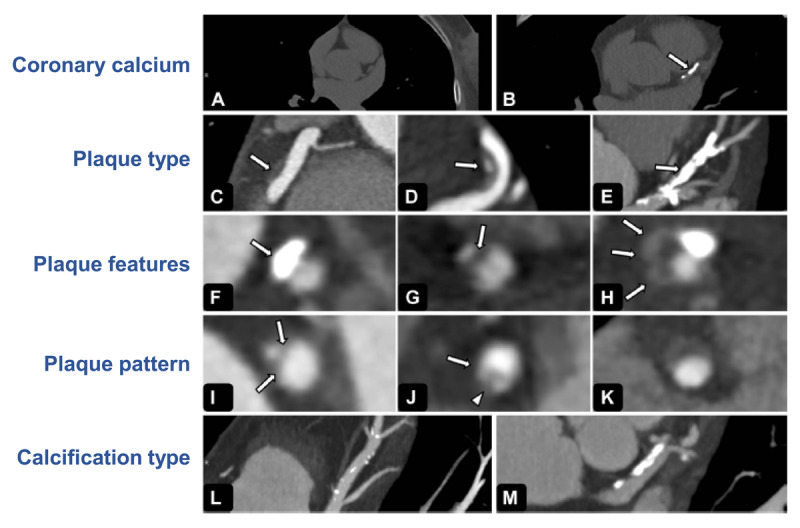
Plaque analysis by CCTA. To assess the (A) absence or (B) presence of coronary artery calcium. Plaque type is classified as noncalcified (C), partially calcified (D), or calcified plaque (E). Plaque features are classified as positive remodeling (panel (F) shows external remodeling of calcified plaque), (G) low-attenuation plaque, and (H) positive remodeling. Plaque pattern refers to noncalcified regions of plaques and is classified as (I) homogeneous, (J) heterogeneous, or (K) napkin-ring sign noncalcified plaque with enhancing ring surrounding a low-attenuation core. Calcification type is classified as (L) spotty (calcified lesions <3 mm) or (M) large (calcified lesions >3 mm). Abbreviation: CCTA, coronary computed tomography angiography. Reprinted from Voros S, Maurovich-Horvat P, Marvasty IB, Bansal AT, Barnes MR, Vazquez G, et al. *J Cardiovasc Comput Tomogr*. 2014;8(6):442–451. https://doi.org/10.1016/j.jcct.2014.08.006 and reused in accordance with the Creative Commons Attribution (CC BY-NC-ND 4.0 DEED) license.
